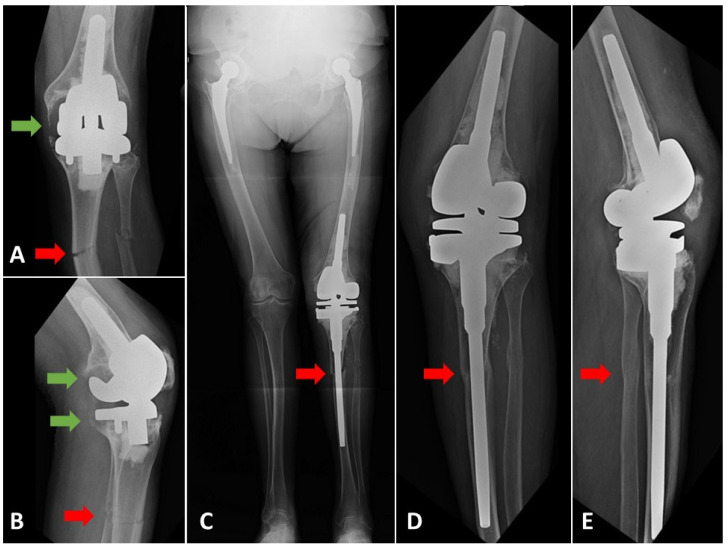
(A) AP and (B) lateral radiographs of a patient with the fracture of 1/3 proximal tibia and fibula shafts and loosened components of the knee prosthesis. (C) Postoperative, long-standing AP radiograph. Rotating-hinge revision knee prosthesis was used (MRH, Stryker, Mahwah, NJ, USA). (D) AP and (E) lateral radiographs of the same patient performed on the control visit 96 months after rTKA. Bone union was achieved. Green arrows indicate peri-implant bone osteolysis and loosening. Red arrows show the fracture level of the tibial shaft and bone union.
